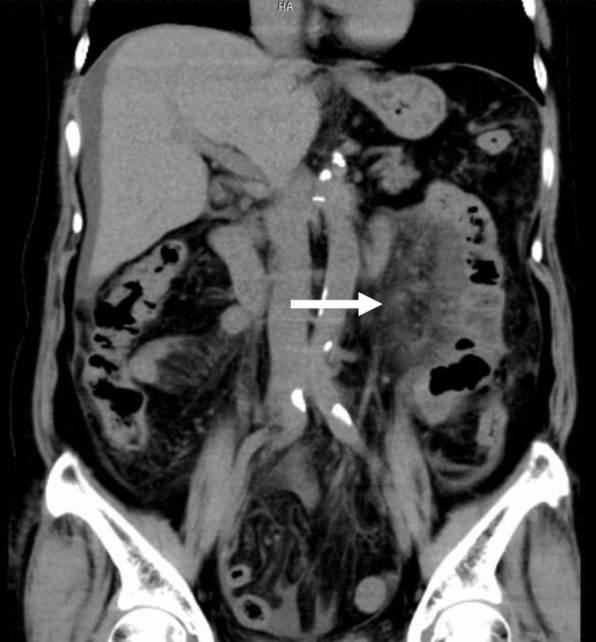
CT image on admission. The thickening mesentery adjacent to the jejunum is showed (arrow). A periarterial halo or stenosis in the superior mesenteric artery is not remarkably visualized
